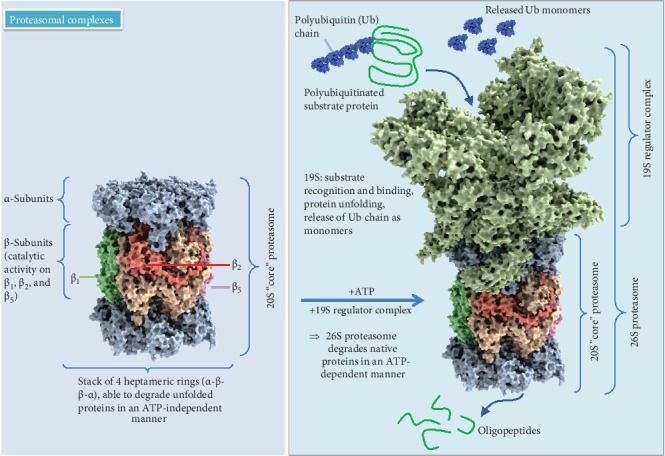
Proteasomal complexes. 20S and 26S models according to data from X-ray structure analysis [267].
